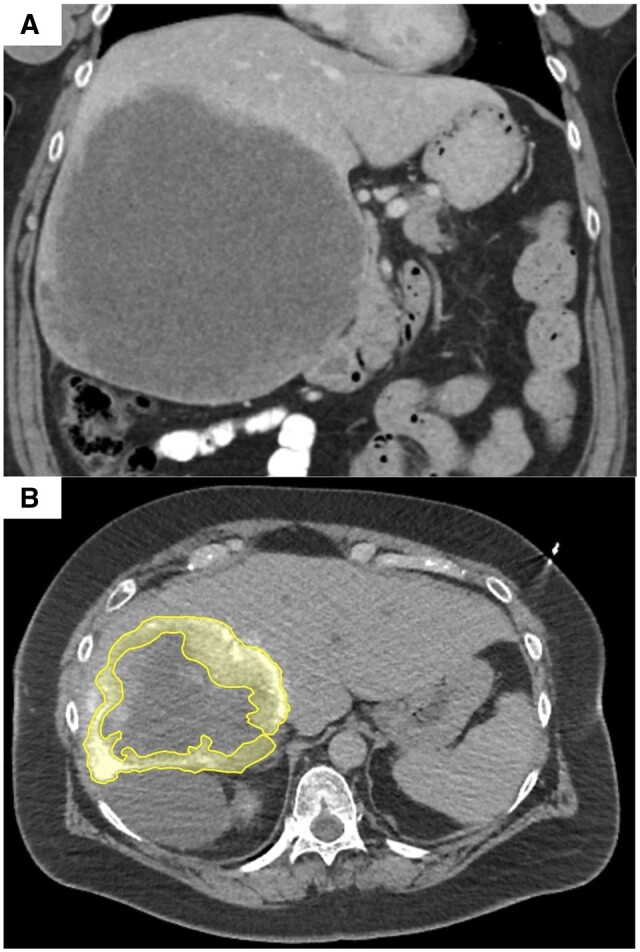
Large areas of central necrosis were major contributors to overestimated volume measurements by the Couinaud anatomic model. (A) Shown in coronal CT images acquired during portal venous phase is a 19.5-cm ovarian metastatic tumour of the entire right lobe. (B) Its corresponding tumoural angiosome, as seen on Angio-CT (yellow area; Patient 23 from Supplementary Table S1), encompasses only a minority of the true tumour volume.
